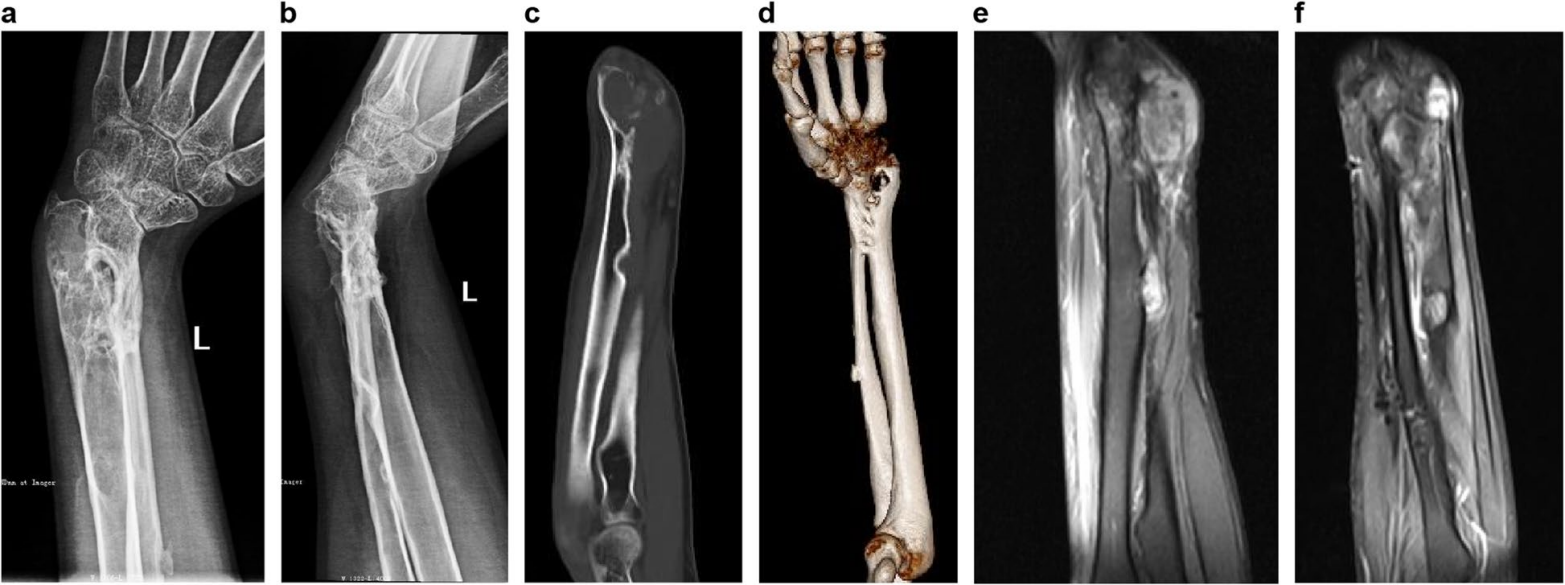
a Anteroposterior and b lateral radiographs show extensive cystic destruction in the distal ulnar with wrist deformity. c Computed tomography (CT) demonstrates expansion and cortical breakthrough of the ulna head accompanied by an extraosseous soft tissue mass. d Three-dimensional CT shows synostosis between the fibula graft and ulna. e, f Magnetic resonance image reveals an irregular mass with an uneven equal signal invading the distal half of the ulna
